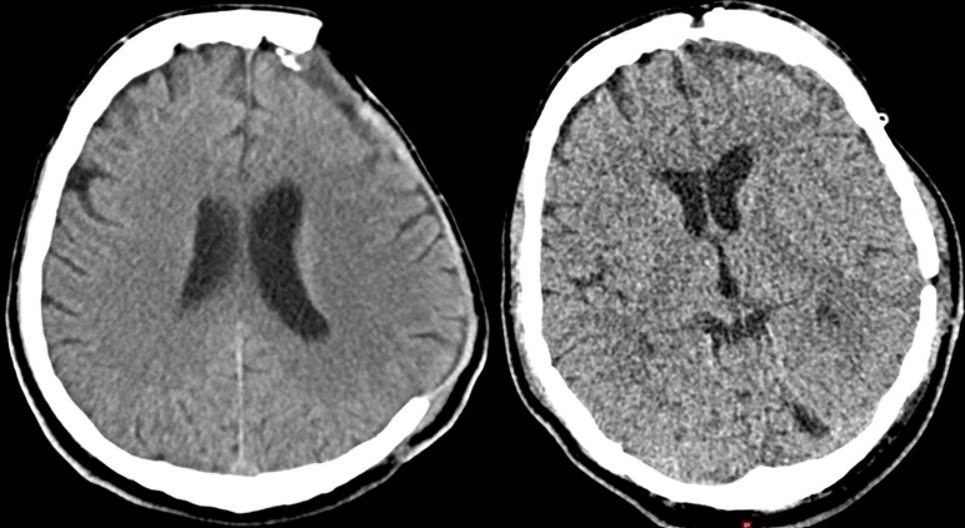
Preoperative and postoperative CT scan of a patient receiving an autologous cranioplasty
